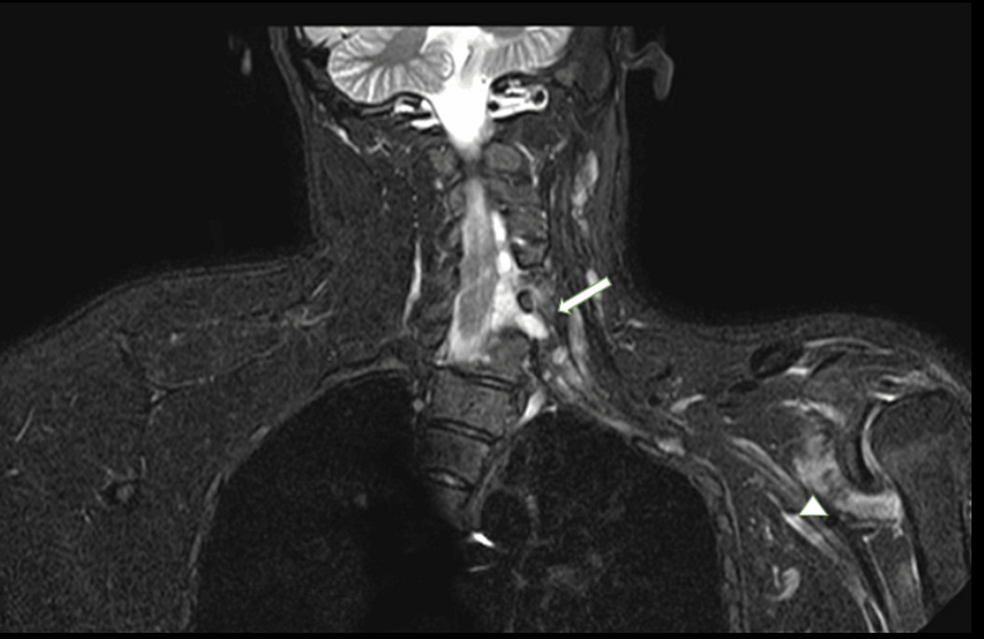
MRI-brachial plexus STIR coronal section of case 2 MRI: Magnetic resonance imaging; STIR: short tau inversion recovery; CSF: cerebrospinal fluid MRI-brachial plexus STIR coronal section showing extradural, CSF-filled collections in the spinal canal (left laterally) (at the levels of C4-C5, C5-C6, and C6-C7 vertebrae) with extension of the CSF-filled structure into the left neural foramen at the levels of C6-C7 vertebrae (large thick white arrow). The left brachial plexus (superior, middle, and inferior trunks in interscalene triangle, six divisions in costoclavicular space, lateral, posterior, and medial cords in retropectoralis minor space) appears as mild edematous (small thick white arrow head).
